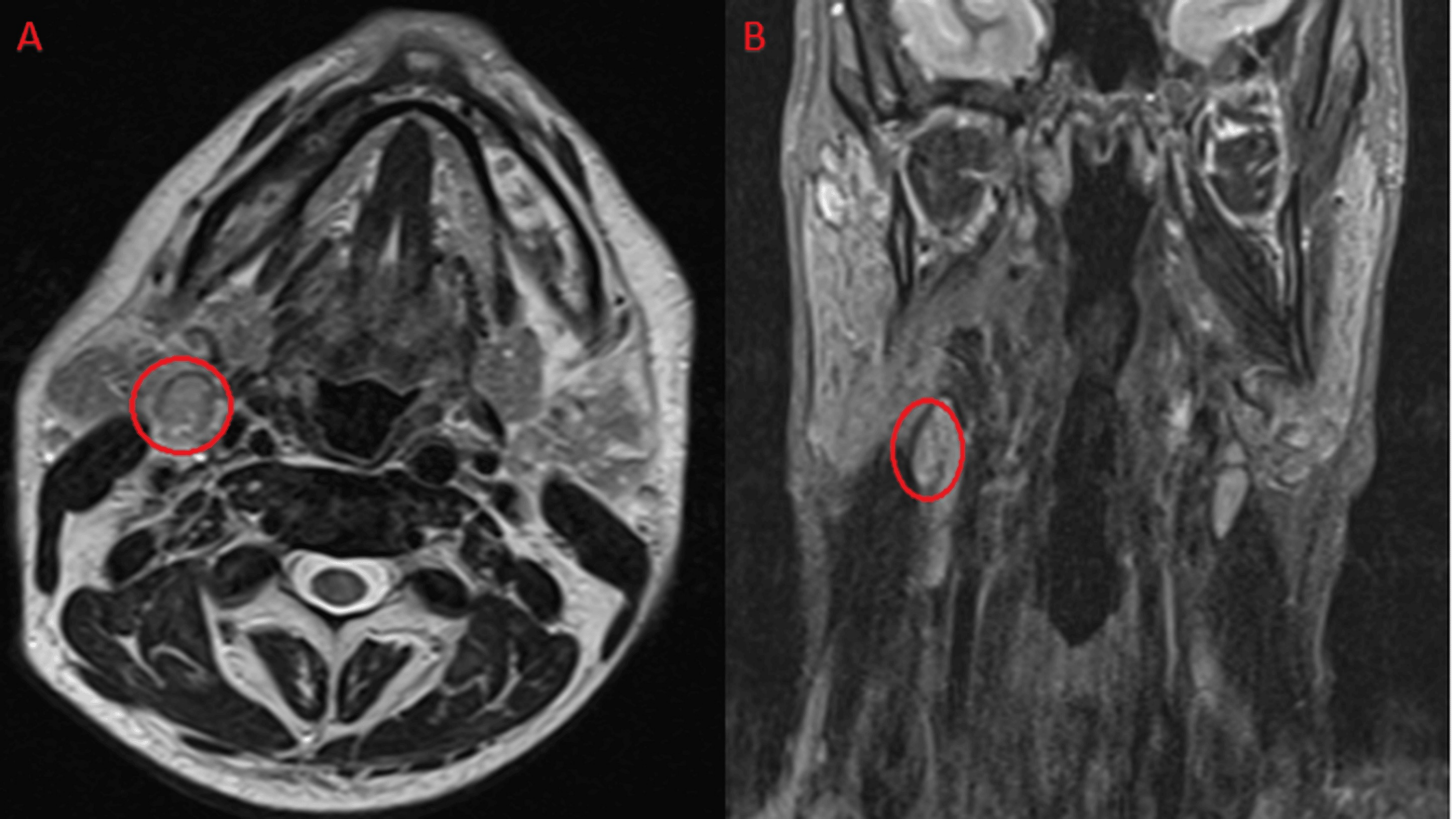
MRI of the neck (A) The red circle shows the right level 2 pathological lymph node axial section T2 sequence MRI image (B). The red circle shows the right level 2 pathological lymph node coronal section T2 sequence MRI image MRI: magnetic resonance imaging
